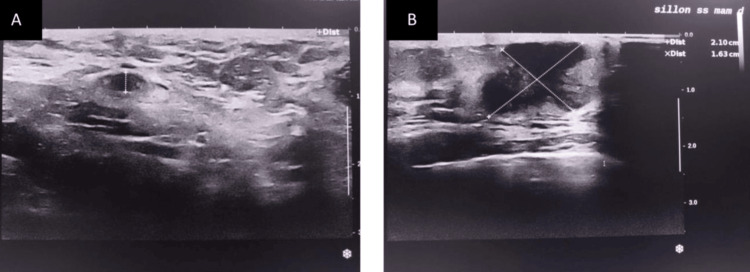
Ultrasound mammography showing bilateral mastoid plaques, a suspicious right retro-nipple lesion, and largely necrotic ipsilateral lymphadenopathy. Classified as ACR BIRADS 5 on the right and BIRADS 3 on the left.
